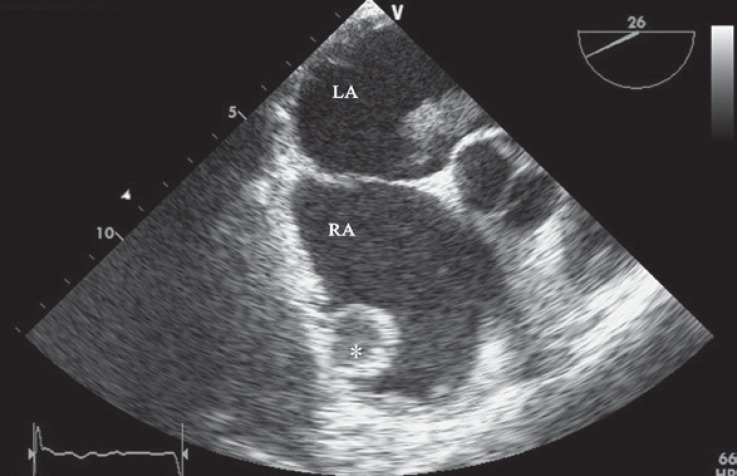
Right atrial thrombosis (*) is depicted in transesophageal echocardiography (upper esophageal view).
